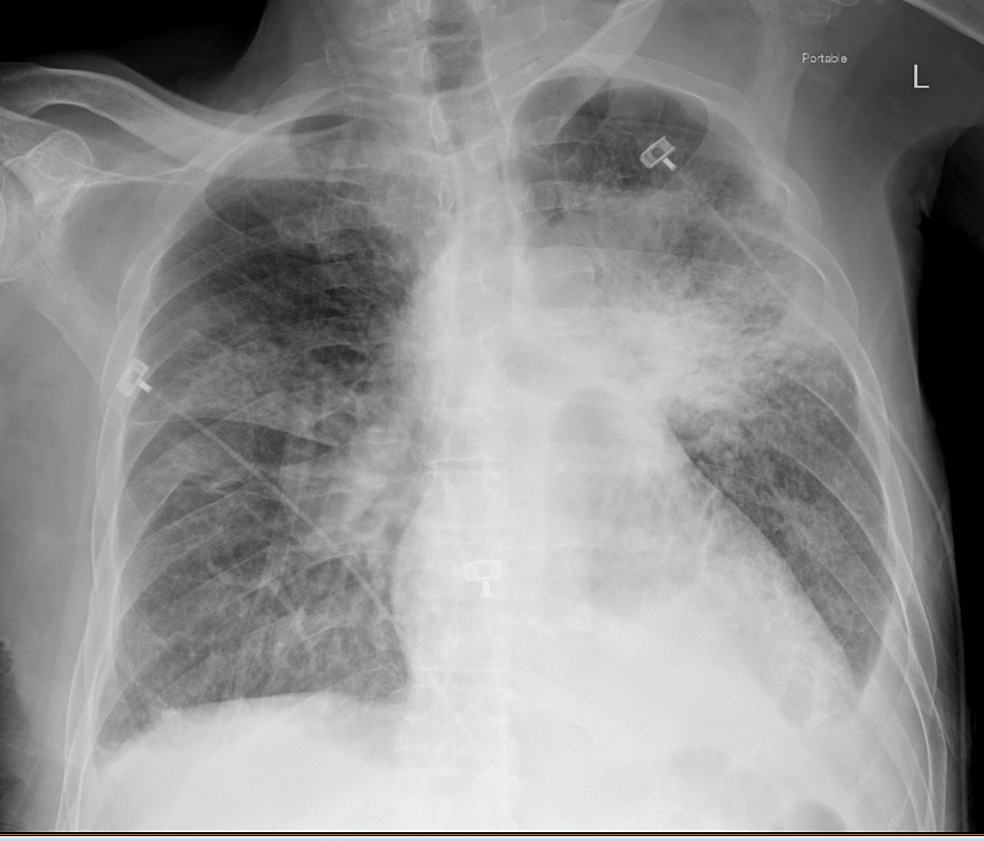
Chest radiography image on admission The image is showing a trace right pleural effusion and a small left pleural effusion, bilateral hazy opacities with normal heart size.
